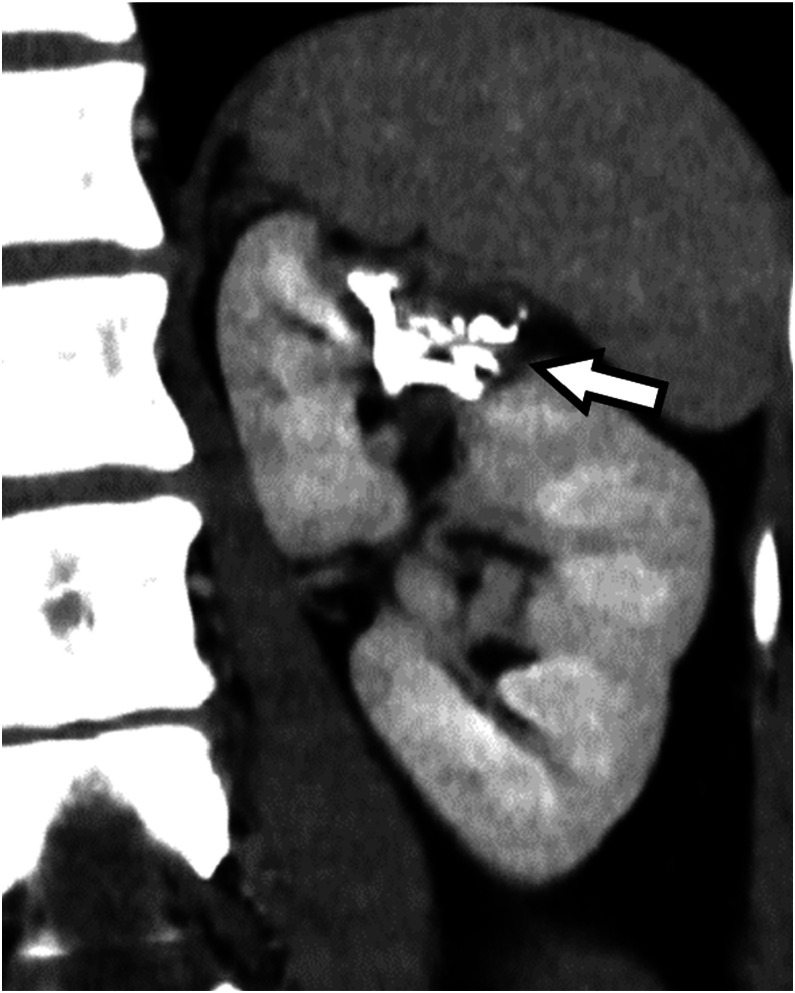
Contrast-enhanced computed tomography image 15 weeks after embolization shows tumor shrinkage, absence of the contrast effect of the tumor, and good contrast effect of the normal renal region. The injected NLI remains in the tumor vessels (arrow).
